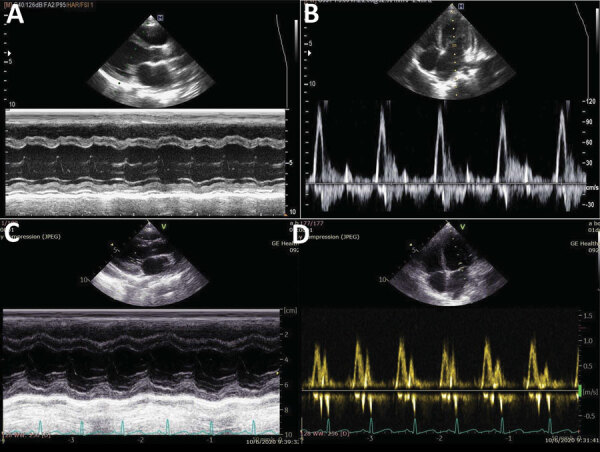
Serial echocardiographic recordings for 7-year-old boy later diagnosed with diphtheria myocarditis, Vietnam, 2020. On day 14 of illness, the M-mode left ventricular ejection fraction decreased to 40% (A) and the E/A ratio was >3.5 (B). At 2-week follow-up after discharge, both left ventricular systolic (C) and diastolic function (D) had recovered.
